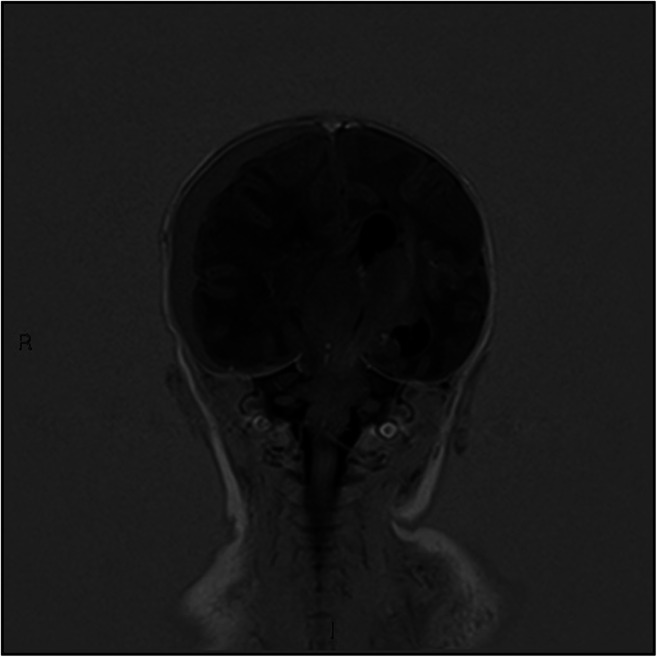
T1-weighted inversion recovery coronal MRI. Large right-sided subdural haematoma with mass effect, midline shift, left-sided hydrocephalus and right-sided uncal herniation
